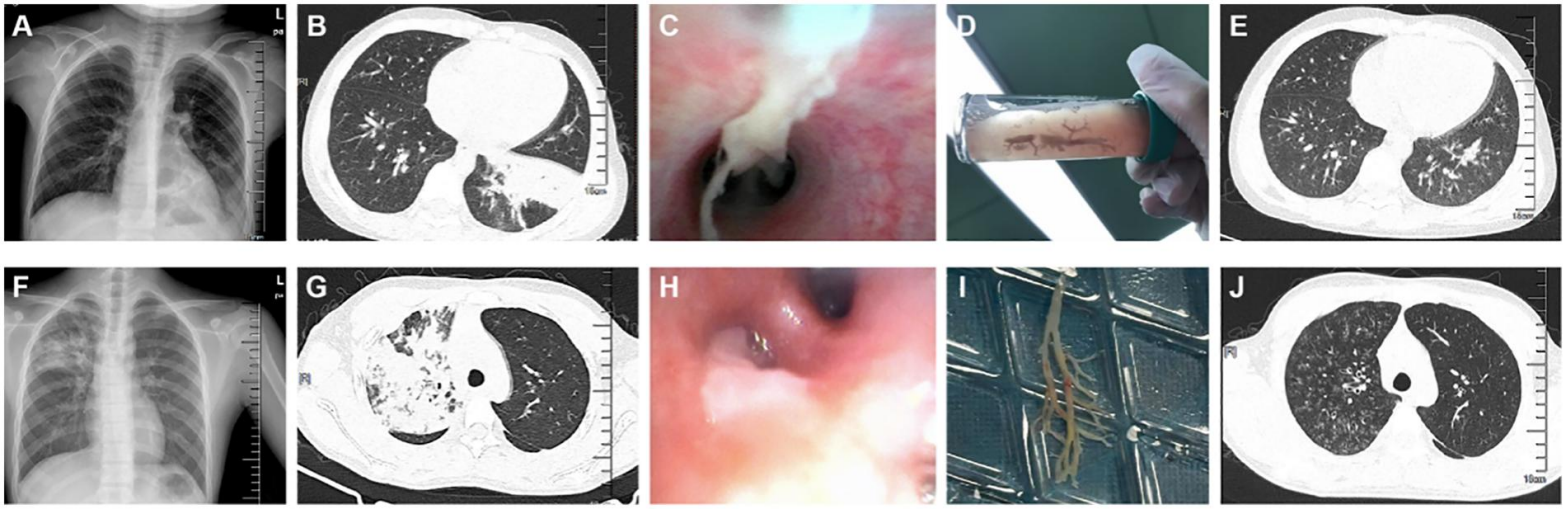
Radiological and bronchoscopic images of plastic bronchitis. Images A–E correspond to a 6-year-3-month-old boy diagnosed with SMPP. (A) Admission chest radiograph shows reticular and patchy opacities with blurred margins in the left lower lung; (B) Chest CT reveals extensive consolidative inflammation in the left lung; (C) Bronchoscopy demonstrates a mucus plug obstructing the left lower lobe bronchus; (D) The PB mucus plug is successfully extracted; (E) Follow-up chest CT at 2 weeks shows near-complete resolution of pulmonary inflammation and full re-expansion of the lung. Images F–J correspond to a 7-year-6-month-old boy diagnosed with SMMP. (F) Admission chest radiograph reveals a large area of increased opacity in the right upper lung; (G) Chest CT demonstrates a high-density shadow with atelectasis in the right upper lobe; (H) Bronchoscopy shows a mucus plug occluding the right upper lobe bronchus; (I) The mucus plug is successfully removed; (J) Follow-up CT at 10 days indicates complete pulmonary re-expansion and near-total resolution of inflammation in the right lung.
